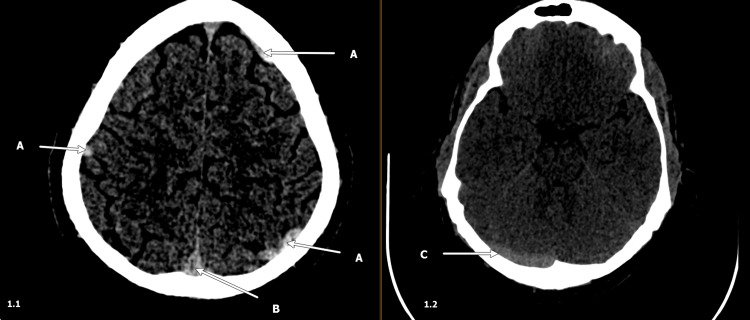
Hyperdense vein signs on non-contrast CT Head. (A) Cortical veins demonstrating hyperdensity due to thrombus. (B) Superior sagittal sinus with hyperdensity. (C) Right transverse sinus demonstrating cord-like hyperattenuation (Cord sign). CT: Computed Tomography
